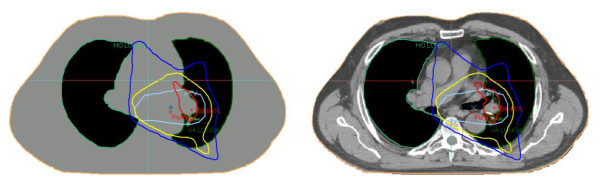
Dose distribution in lung for synthetic and normal CT. The dose distribution in the thoracic area in a bulk density based-treatment plan (left) and in a CT-based treatment plan (right). The PTV is light blue, the 70% isodose is blue, 95% is yellow, and 105% is red.
